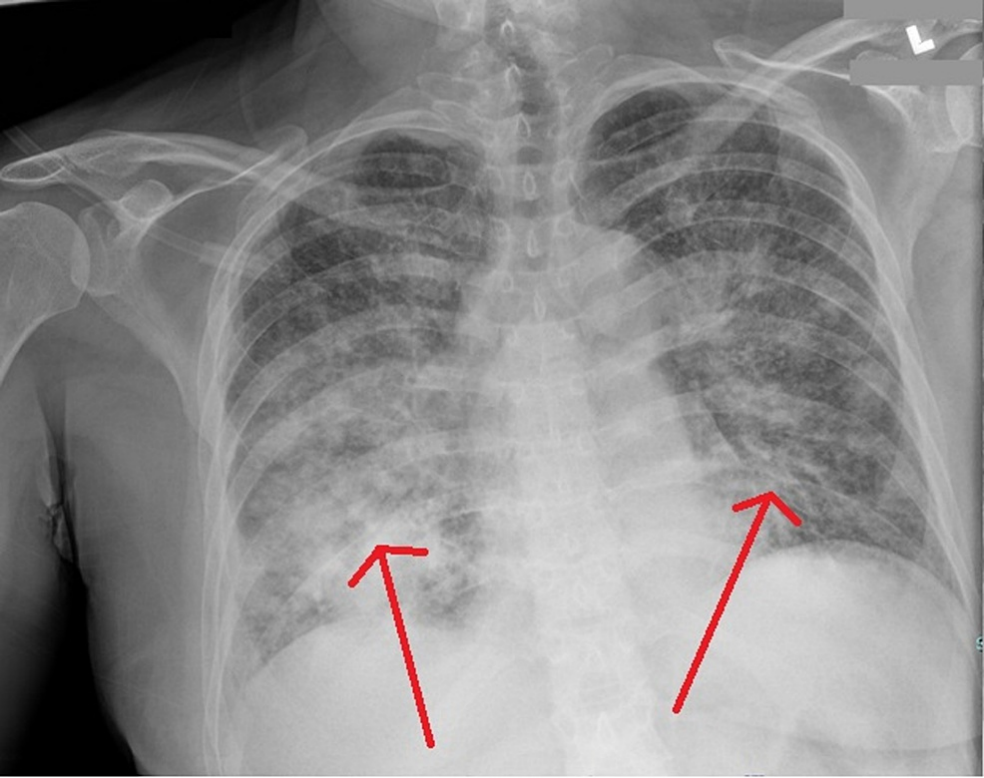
Chest X-ray Portable Anteroposterior (AP) Arrows show bilateral consolidation and nodular opacities, right greater than left, which may represent some combination of confluent metastatic disease, pneumonia, and pulmonary edema. The heart size is normal. Mediastinal fullness is concerning for adenopathy. There is a small right pleural effusion. No left pleural effusion. No pneumothorax.
